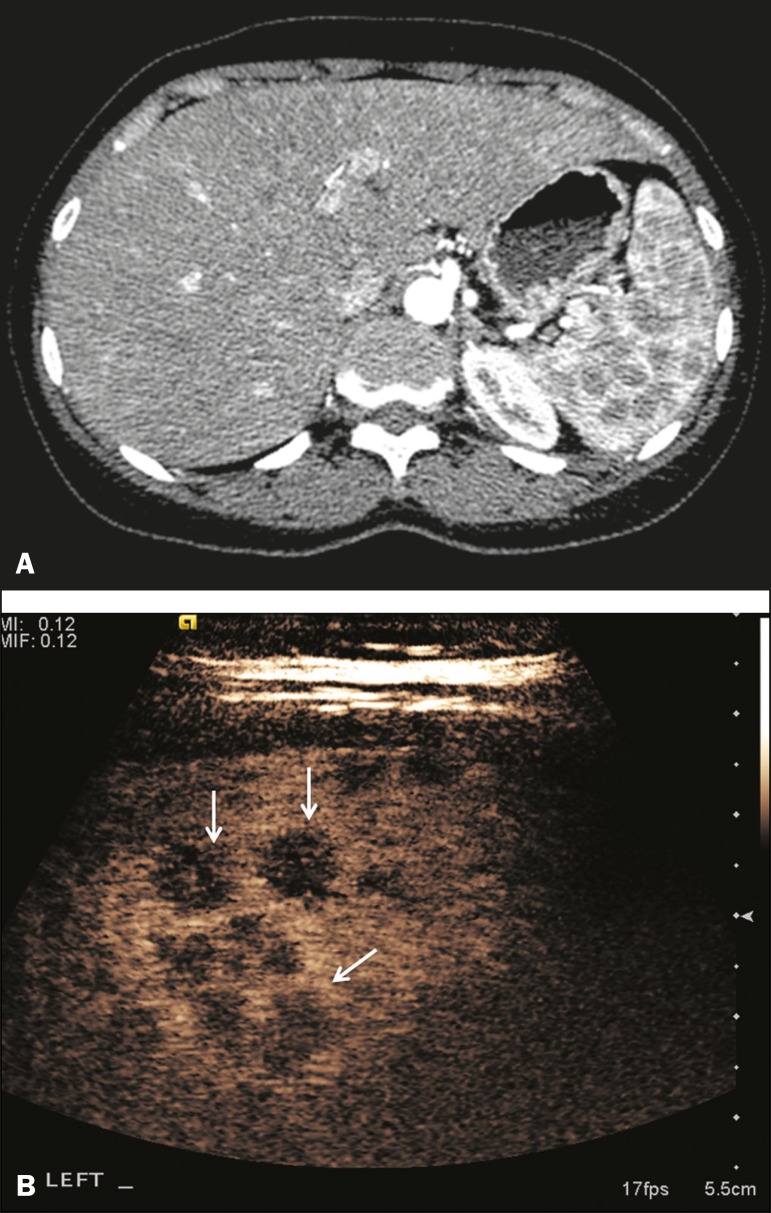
Granulomatous disease. A: Contrast-enhanced CT scan
